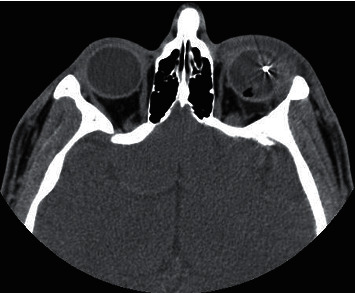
Open globe injury in a 34-year-old man. Axial unenhanced CT image showing a metallic intraocular foreign body.
